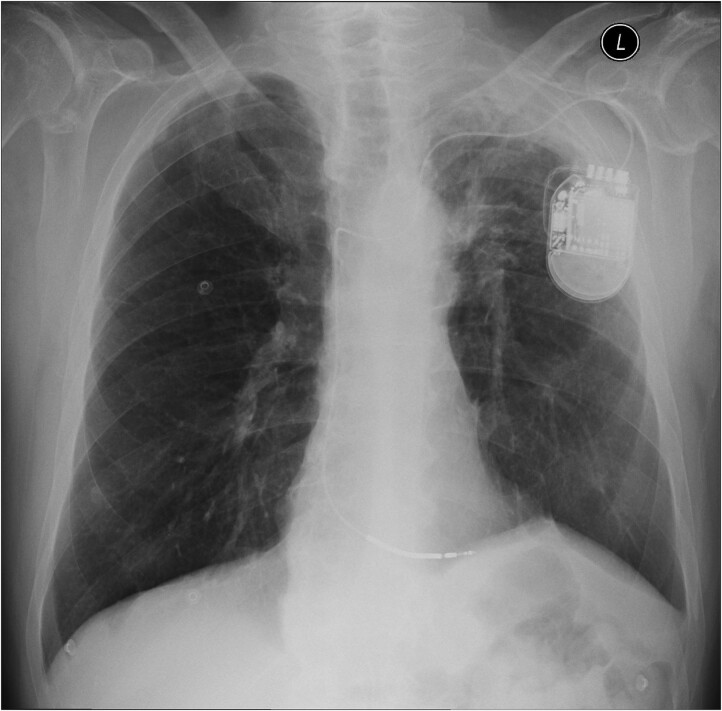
Chest X-ray (anteroposterior view) after the first implantation.
